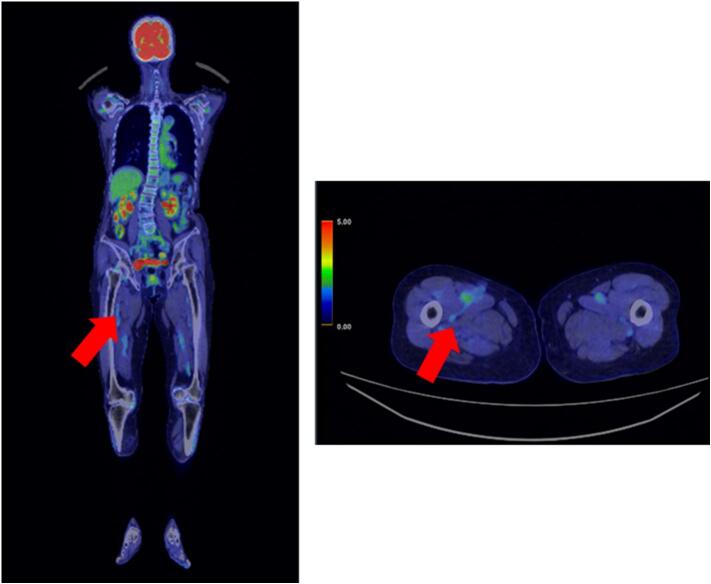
PET-CT scan 3 years postoperatively, PET-CT showed no detectable tumor lesion on the medial side of the right thigh (arrow) and no metastasis.
